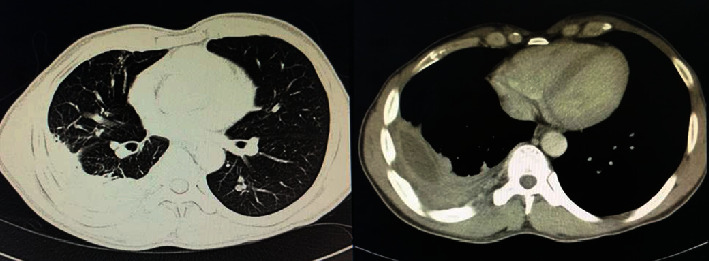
Contrasted enhanced CT thorax revealed a multiloculated collection within the right hemithorax depicting the thickened enhancing wall which joins at the margins of the collection to form the split pleura sign. This is associated with the compression atelectasis surrounding it.
